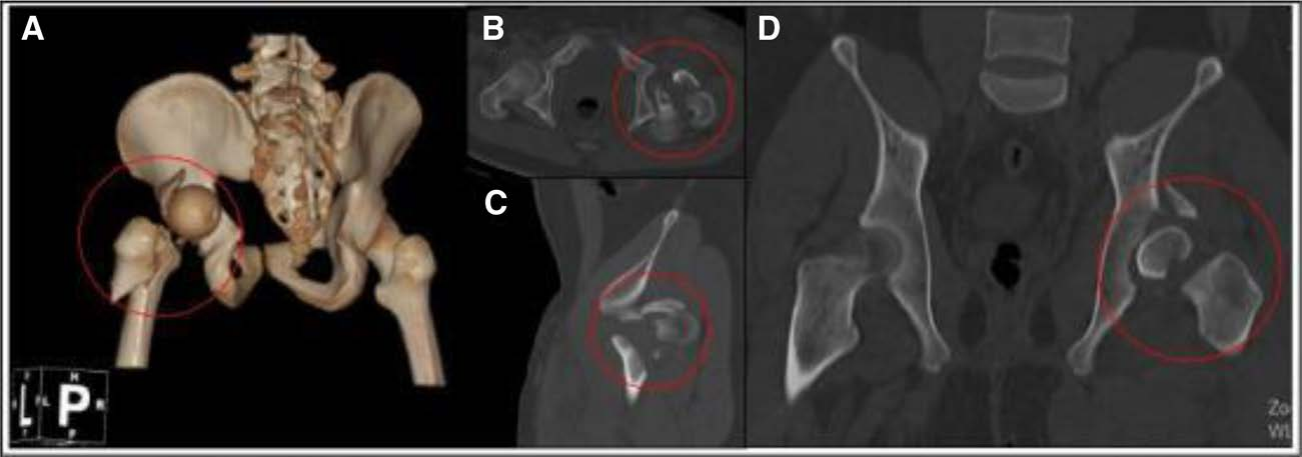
The patient preoperative radiographs and computed tomography images showed ipsilateral femoral intertrochanteric, femoral neck, and acetabular fractures with posterior dislocation of the femoral head. (A) Preoperative pelvic CT 3-dimensional reconstruction; (B) preoperative cross-sectional CT image of the hip joint; (C) preoperative sagittal CT image of the hip joint; (D) preoperative coronal CT image of the hip joint. CT = computed tomography.
